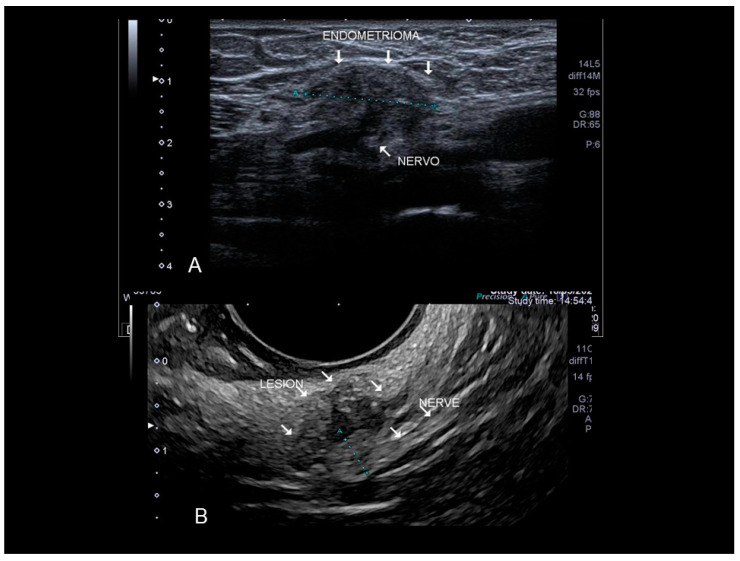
Endometriosis of very lateral retroperitoneal implant (see white arrow) in the topography of Latzko space with nerve involvement confirmed at surgery. The clearest portion measured probably refers to the compromised epineurium. Findings confirmed by MRI and electroneuromyography (A). The last picture is a nerve branch involvement by wall endometrioma in the topography of external iliac vessels (see white arrow) (B). Confirmed by surgery.
